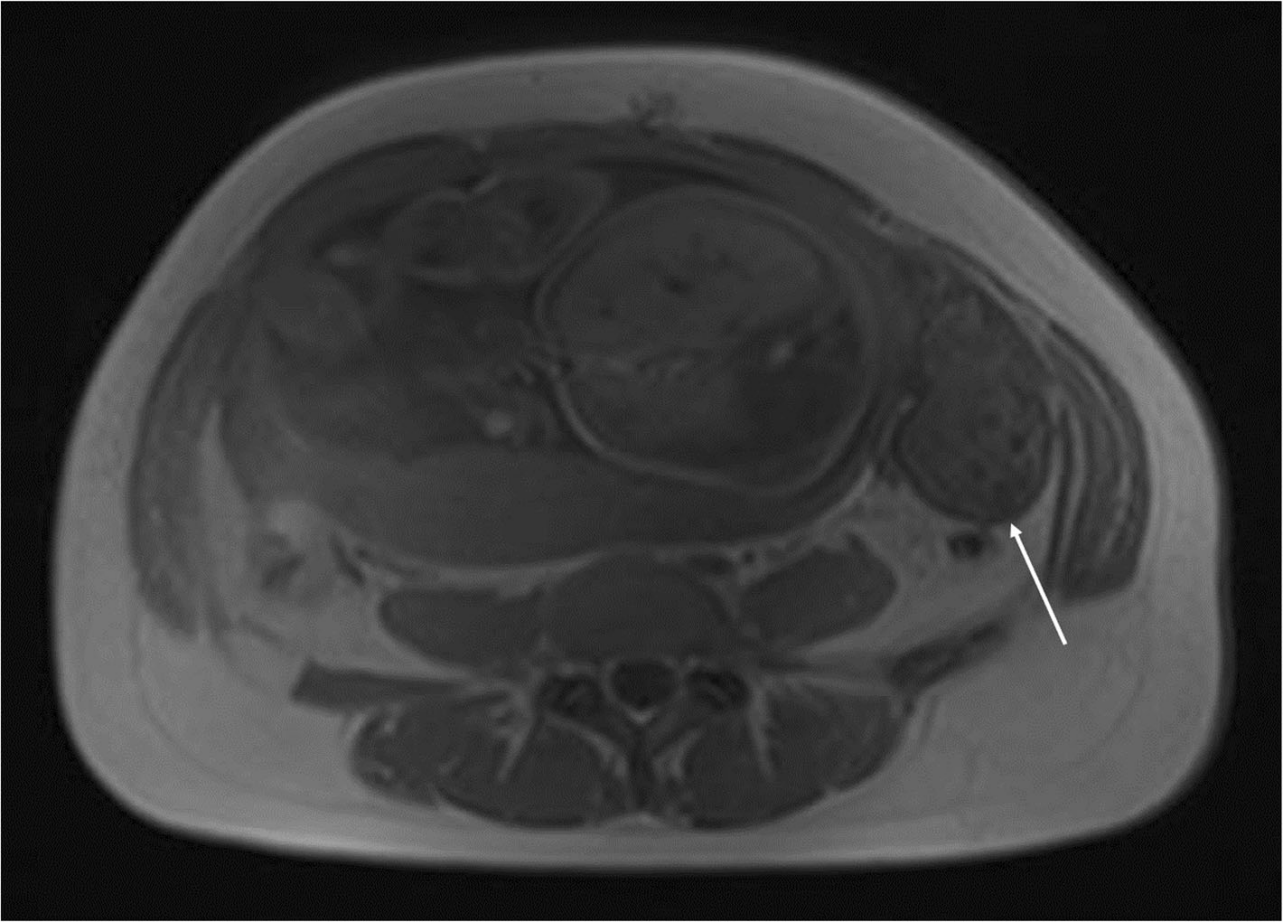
Axial fat-saturated T1 weighted magnetic resonance imaging (MRI) shows the enlarged left ovary had a signal intensity equal to that of muscle but was not homogeneous (long arrow)
